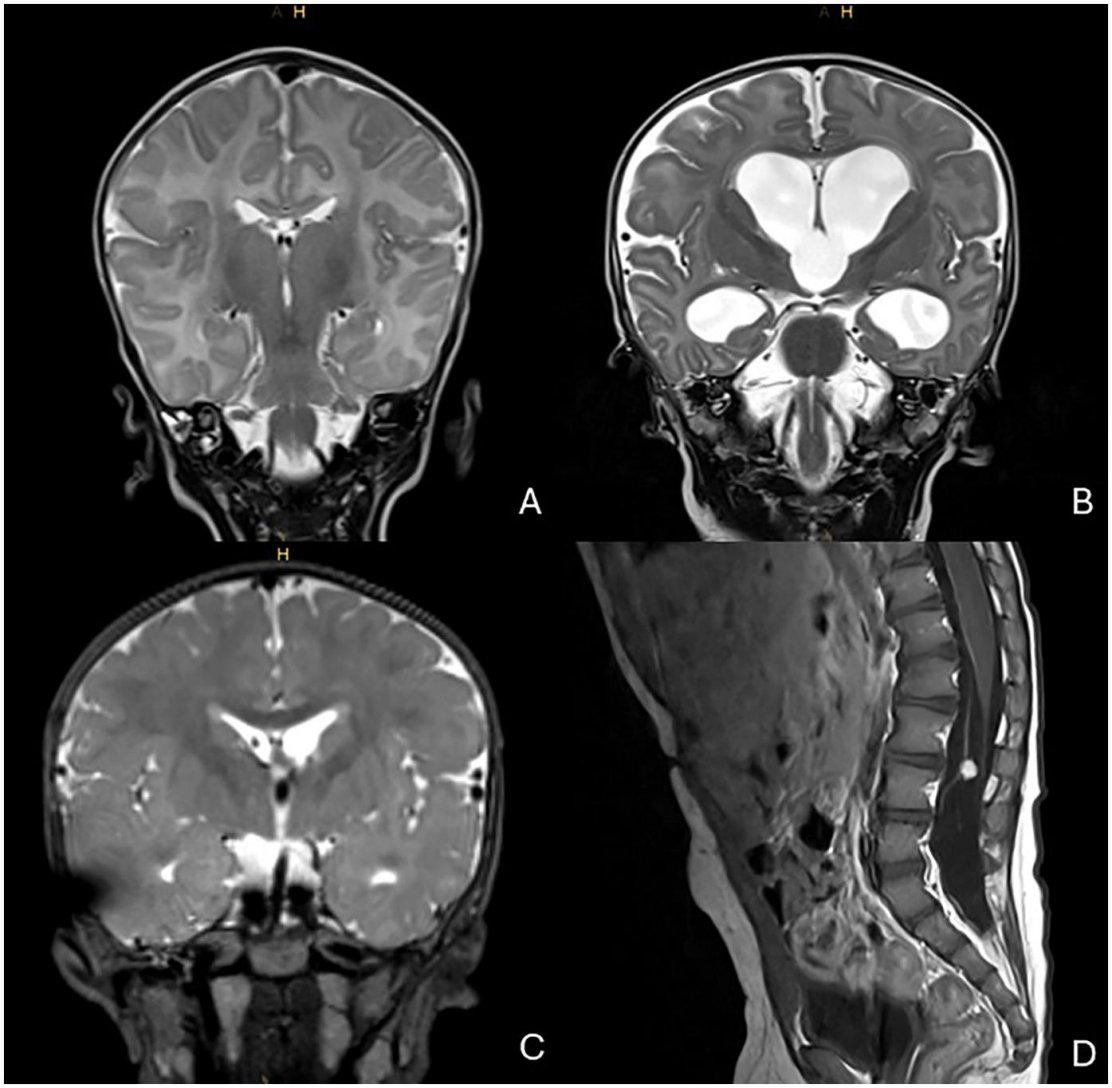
MRI imaging over time. (A) T2-weighted MRI during initial hospitalization (1 month, 17 days of age); normal cerebral ventricular width. (B) T2-weighted MRI at 6 months, 18 days of age; hydrocephalus with enlargement of ventricles I–III. (C) T2-weighted MRI at 1 year, 24 days of age; relief of hydrocephalus via right frontal shunt catheter. (D) T1-weighted MRI with contrast at 1 year, 10 months of age; solid nodule with contrast enhancement, stable in size since initial detection at 1 year, 24 days of age.
